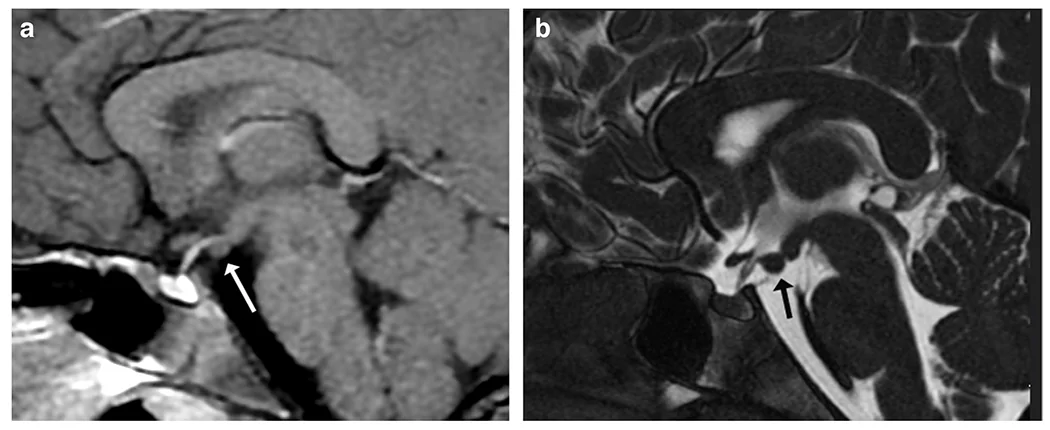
Six-year-old female with central precocious puberty and parahypothalamic (pedunculated) hamartoma. a Sagittal T1-weighted post-contrast and (b) sagittal volumetric T2-weighted steady-state acquisition images show a non-enhancing and uniformly isointense nodule of tissue positioned tangential to the inferior surface of the hypothalamus (*white arrow* in a, *black arrow* in b)
